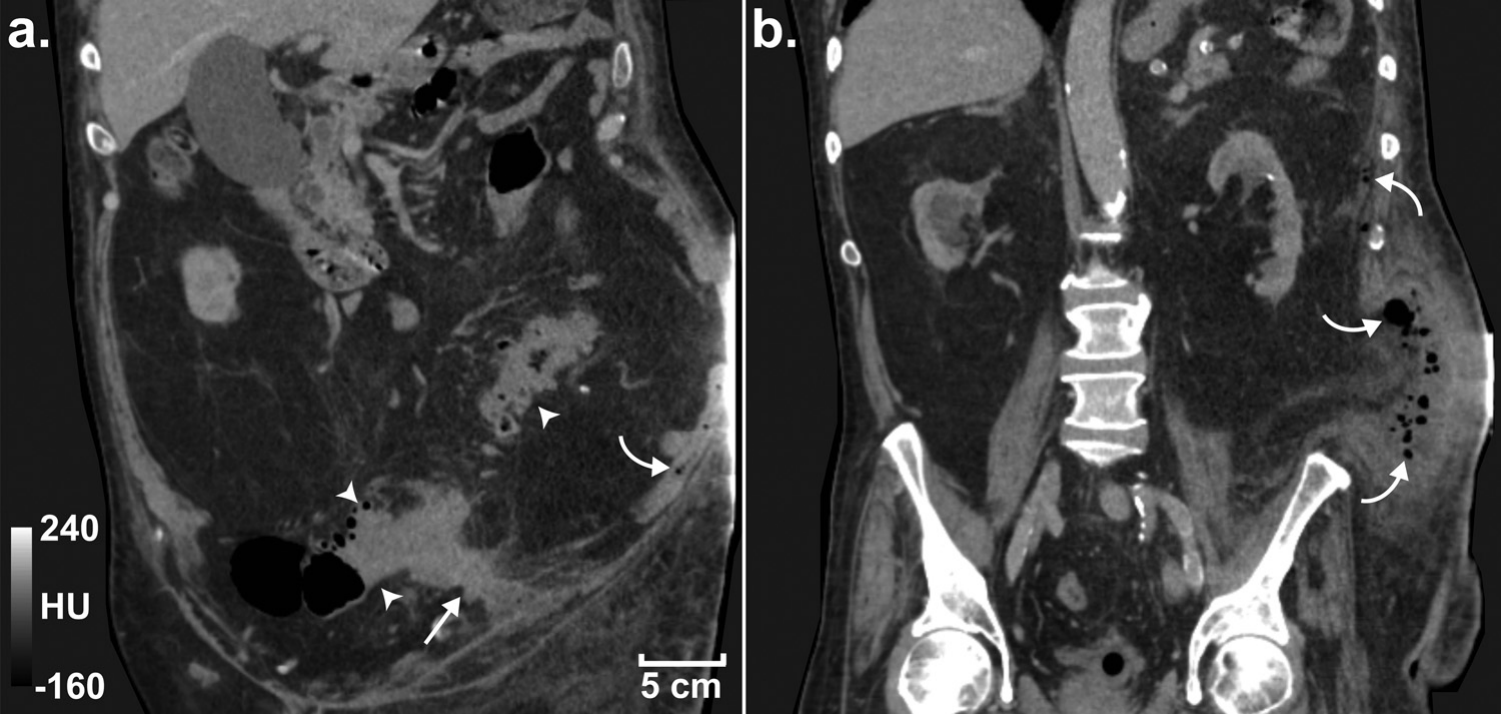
Admission CT images demonstrating the abscess forming a sinus tract between the sigmoid colon and retroperitoneum. (A) Coronal slice demonstrating sigmoid diverticulitis (arrowheads), the abscess/sinus (arrow) and locules of free gas (curved arrow) in the retroperitoneum. (B) A more posterior coronal slice demonstrating the extent of the free gas and fluid in the retroperitoneum. All images have a window width and level of 400 and 40, respectively, and a spatial resolution of 0.63 × 0.63 × 3 mm.
